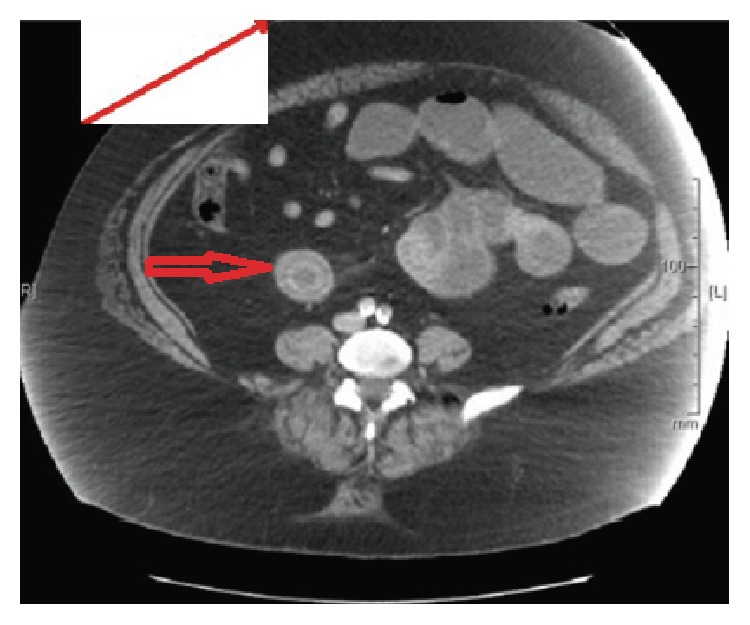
Contrast CT scan depicting gallstone in bowel (short red arrow).
